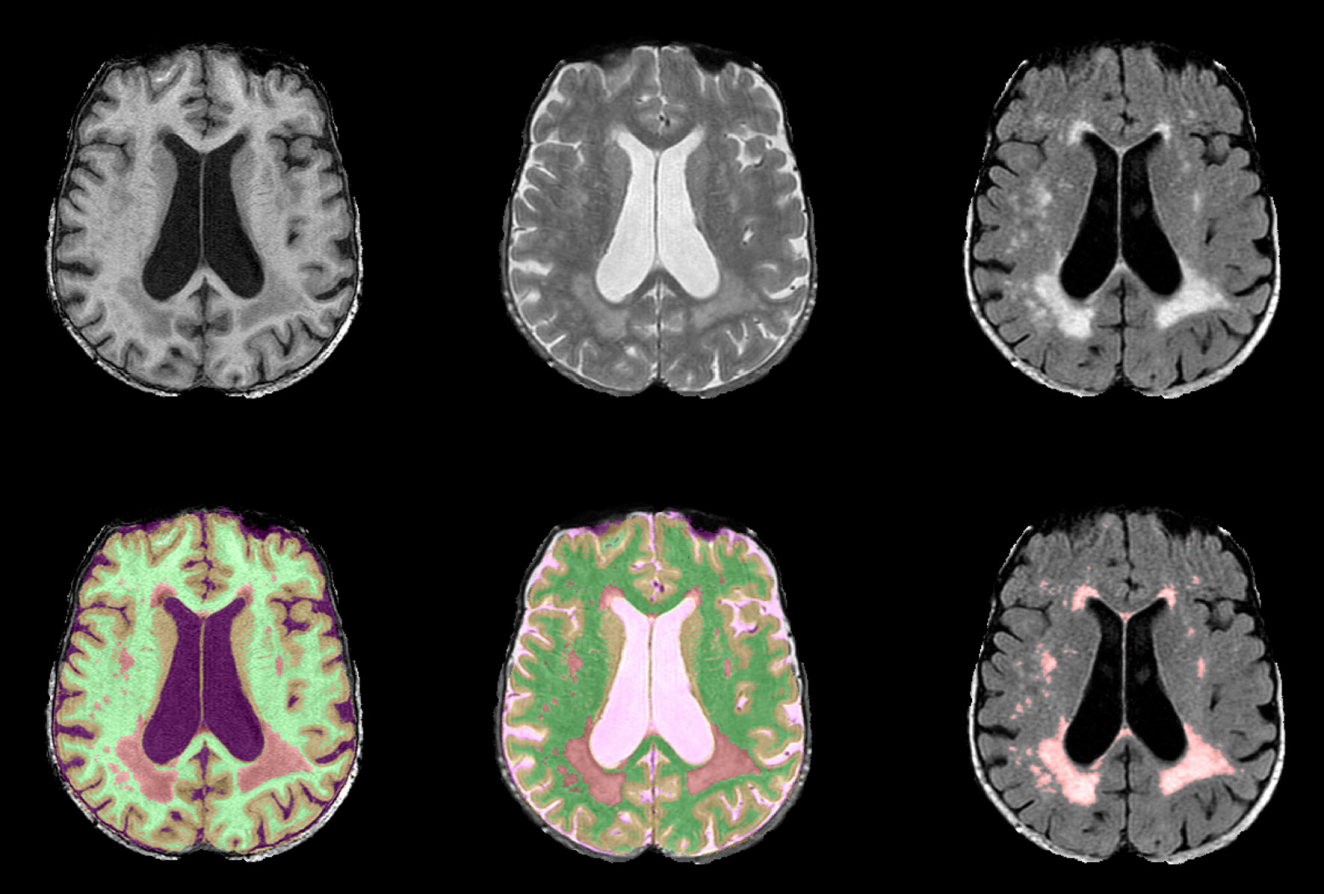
Example of brain tissue segmentation. *Left column* T1weighted sequence with k-nearest neighbor based tissue segmentation into grey matter (*orange*), white matter (*green*), cerebrospinal fluid (*purple*), and white matter lesions (*red*). Middle column: Proton-density weighted sequence with similar tissue segmentation. *Right column* FLAIR-sequence with white matter lesion segmentation (*pink*)
